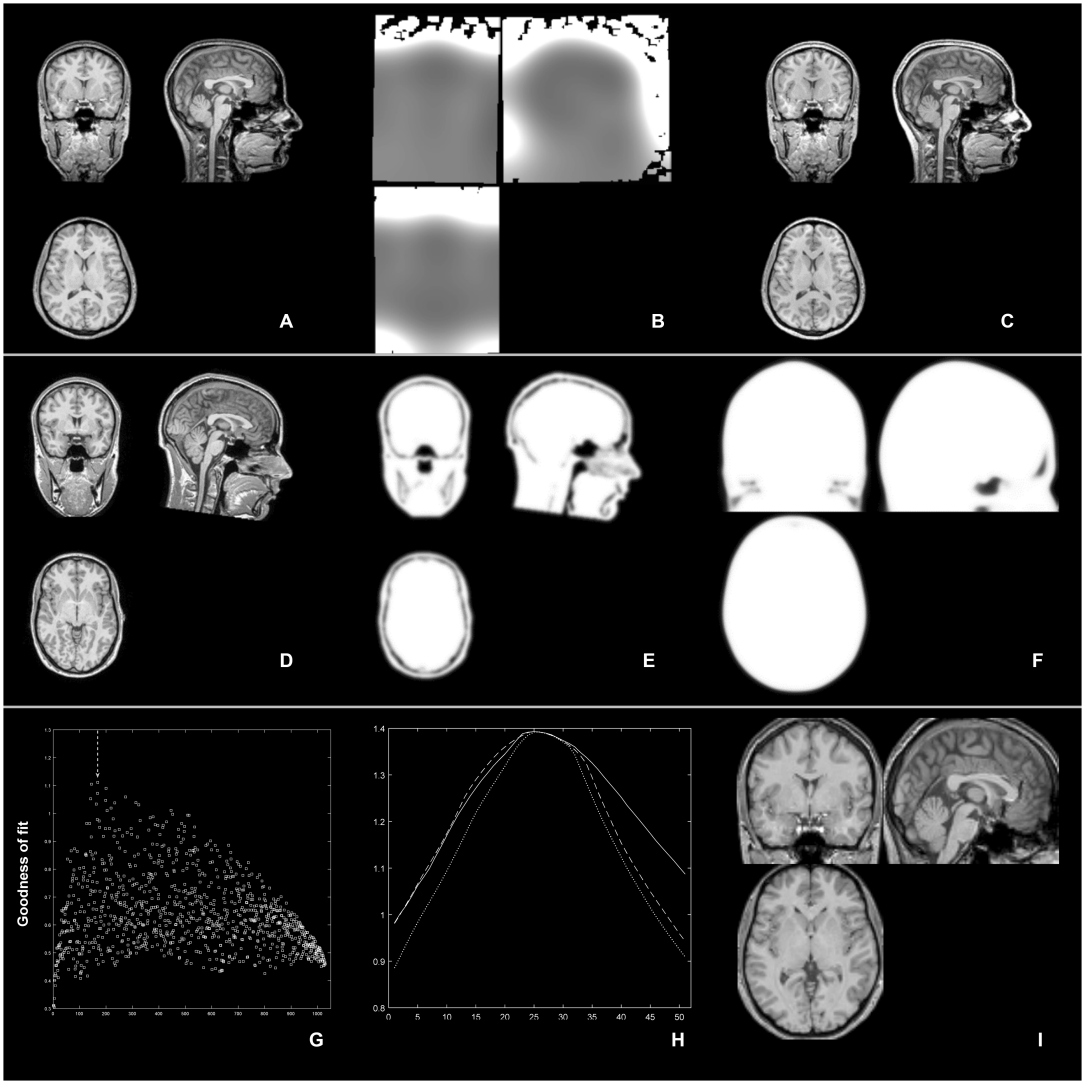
Overview of the 3 step approach: top row (inhomogeneity removal): from an MR image (A), image inhomogeneities are removed (B) and the bias-corrected image (C) is then used to derive the affine transformation parameters; middle row (complexity-reduced image): an MR image (D) is binarized and smoothed (and thus, complexity-reduced; E) and is then matched to a similarly-reduced template (F); bottom row (iterative processing): from an initial, unbiased exploration of the whole search space, the best of 1,000 combination is identified (highest “goodness of fit” value in G, arrow); thereupon, each scaling parameter (in *X*, *Y*, and *Z*) is further refined in 51 smaller steps (H) to finally yield an optimized parameter set for affine spatial normalization (I).
